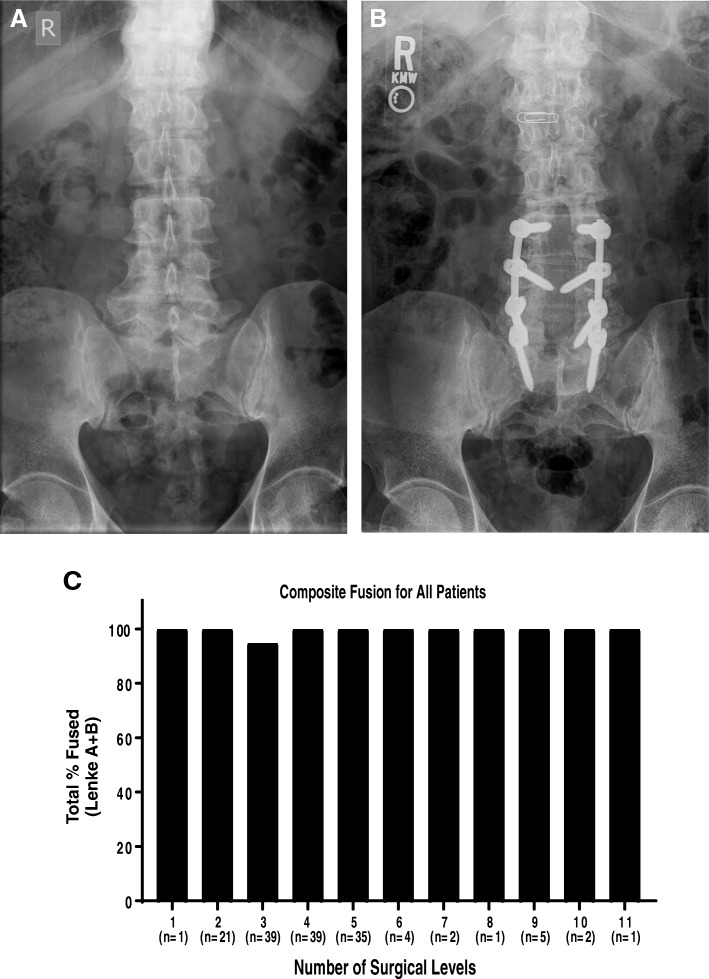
Representative radiographs and combined fusion for all patients. a 69-year-old male, former smoker with a body mass index of 26 presented with back pain and left lower extremity radicular pain. Conservative treatment included physical therapy, stretching, epidural spinal injections, and NSAIDs. b Seven months after L4-S1 decompression and fusion were performed, the patient was fused (Lenke A). c Combined fusion graph (Lenke A + Lenke B) shows that 148 of 150 patients (98.7%) were considered fused: 119 patients Lenke A and 29 Lenke B
